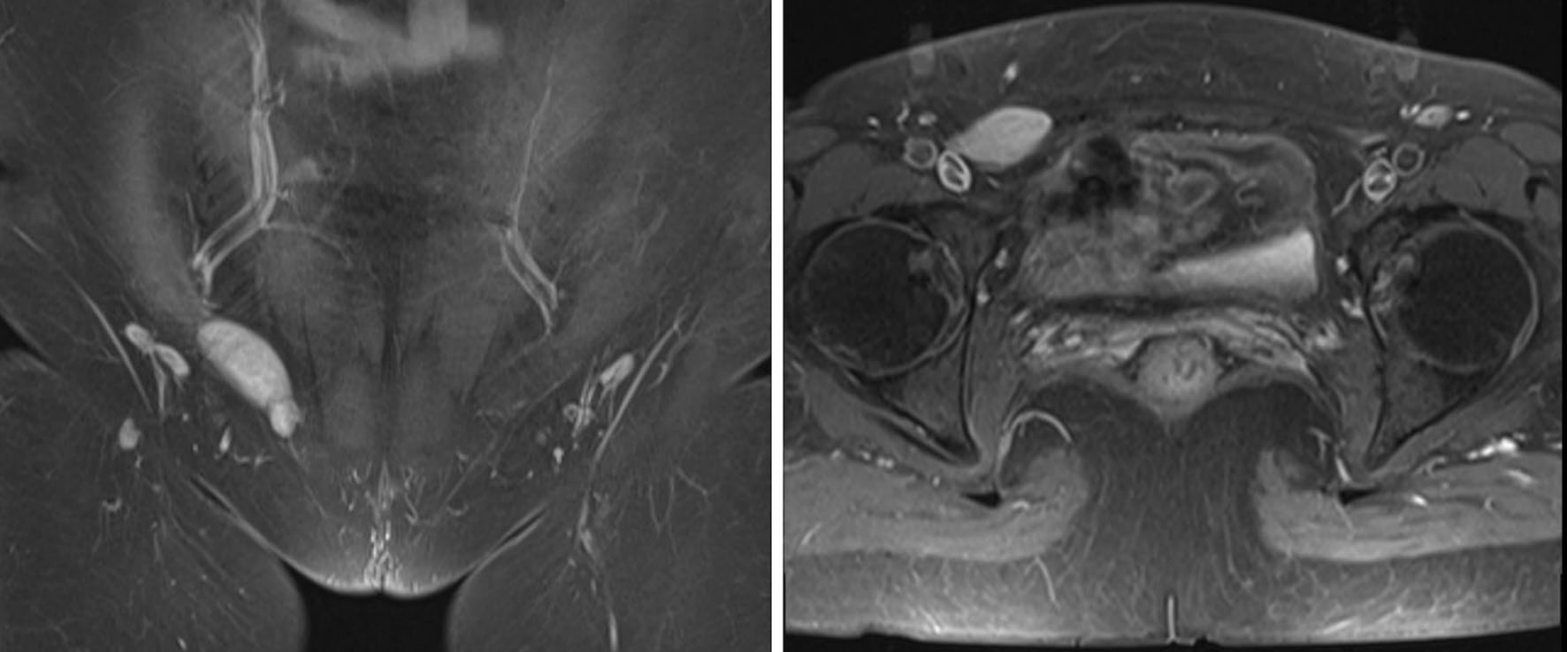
Enhanced magnetic resonance imaging showed that an intermediate enhanced mass located in right inguinal canal
